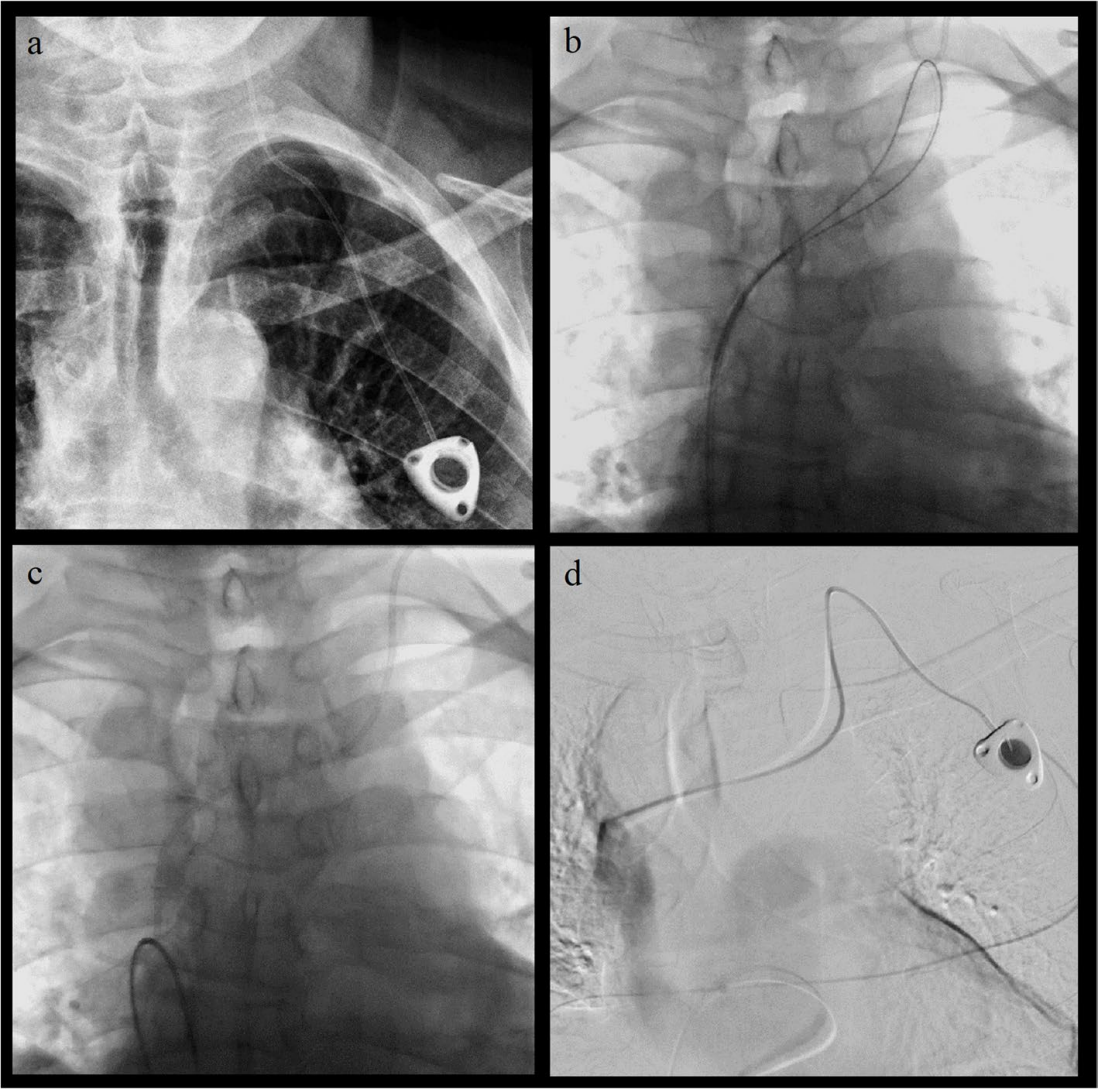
Clinical sample case. a X-ray showing the port systems inserted in the left jugular vein with catheter dislodged high in the ipsilateral jugular vein. b Fluoroscopic image of the long loop with partial reposition of the port catheter in the innominate vein. c Fluoroscopic image with almost complete reposition of the port catheter. d Digital subtraction angiography proving the functionality of the port system
